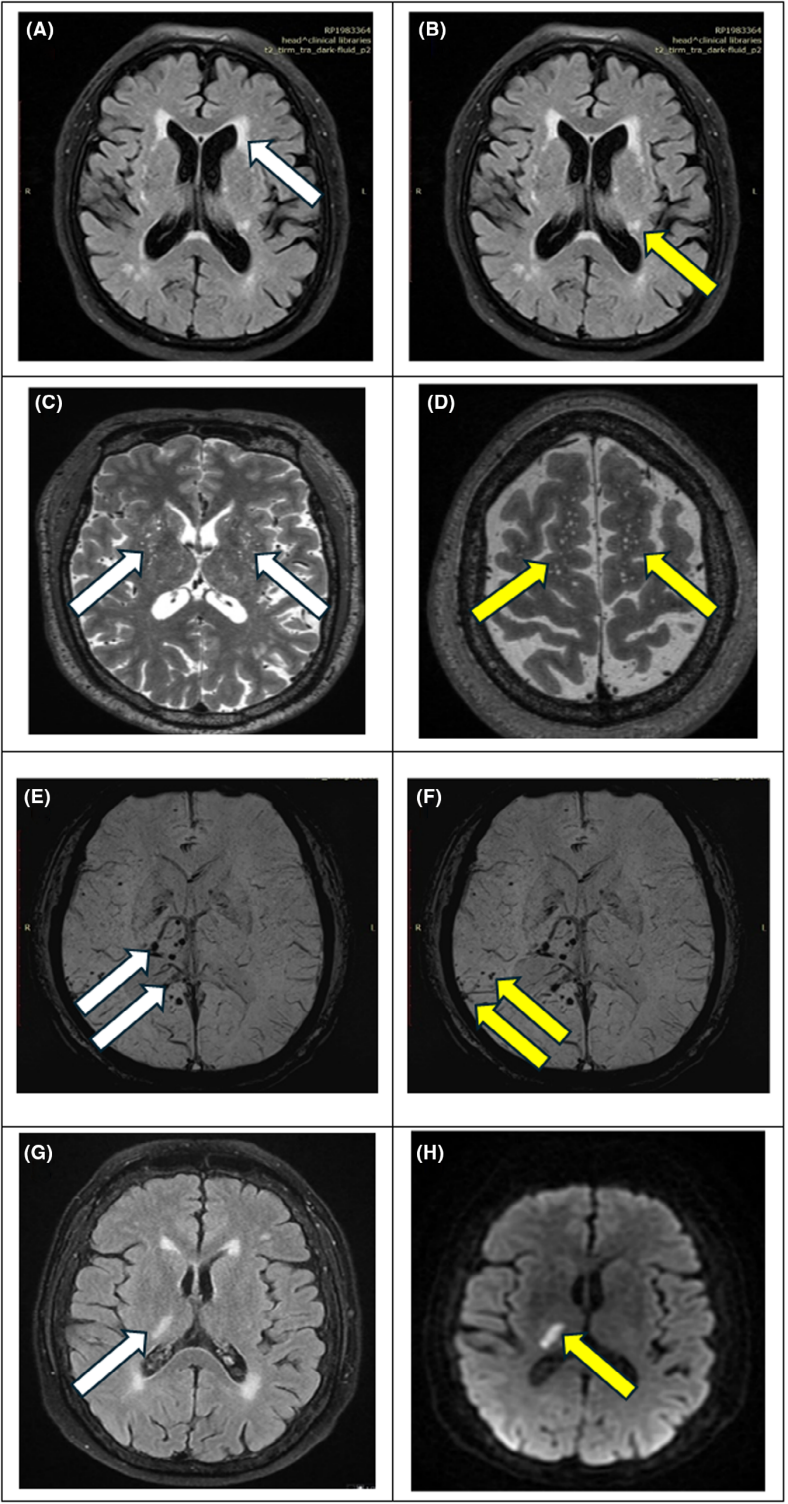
(A) Axial brain MRI on FLAIR sequence showing periventricular white matter hyperintensities (white arrow). (B) Axial brain MRI on FLAIR sequence showing deep subcortical white matter hyperintensities (yellow arrow). (C) Axial brain MRI T2‐weighted sequence showing perivascular spaces in the basal ganglia (white arrows). (D) Axial brain MRI T2‐weighted sequence showing perivascular spaces in the centrum semiovale (yellow arrows). (E) Axial brain MRI susceptibility‐weighted imaging sequence showing deep cerebral microbleeds (white arrows). (F) Axial brain MRI susceptibility‐weighted imaging sequence showing lobar cerebral microbleeds (yellow arrows). (G) Axial brain MRI FLAIR sequence demonstrating an acute infarct in the posterior limb of the right internal capsule (white arrow). (H) Axial brain MRI diffusion‐weighted imaging sequence demonstrating an acute infarct in the posterior limb of the right internal capsule (yellow arrow).
